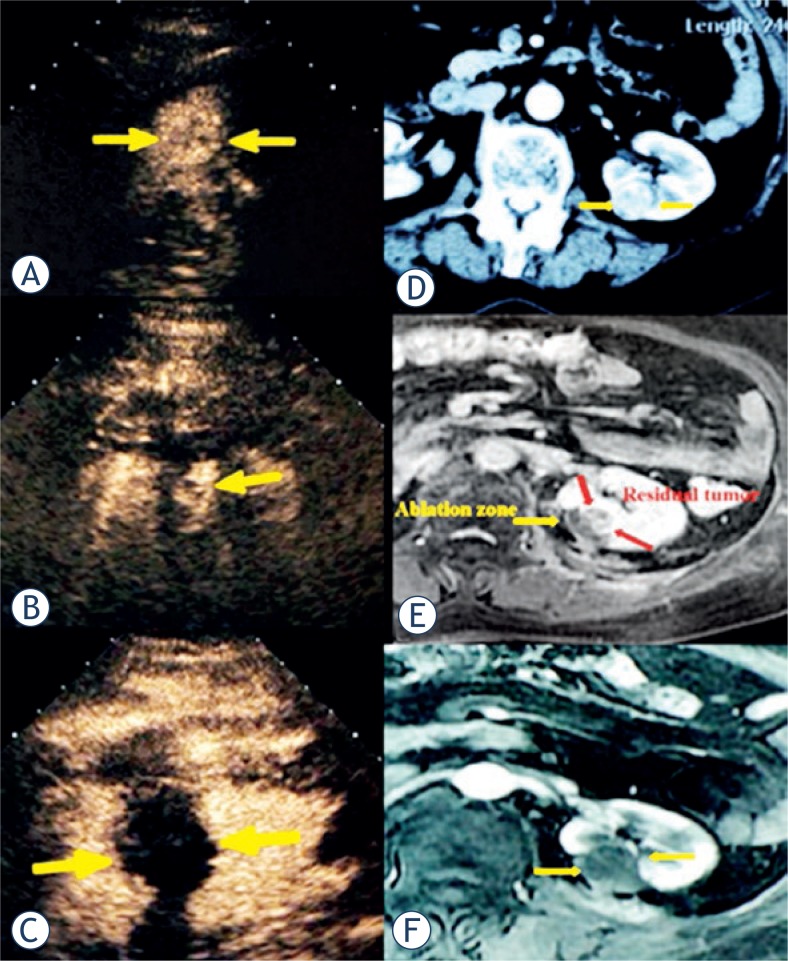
72-year-old female with a 4.5 cm × 3.9 cm renal clear cell carcinoma was treated with microwave ablation (MWA). (A) Pre-ablation contrast-enhanced US scan showed one hyper-enhancement lesion (arrow), (B) and 3 days after ablation showed a very small hyper-enhancement adjacent to the ablation zone. (C) Then the patient received another ablation and reached completed necrosis. Transverse contrast-enhanced MR imaging showed one hyper-intense lesion a little exophytic and adjacent to renal pelvis in arterial phase pre-ablation and showed the same hyper-intense (residual tumour) (red arrow) homochronously (D), then no-intense after another ablation (F).
